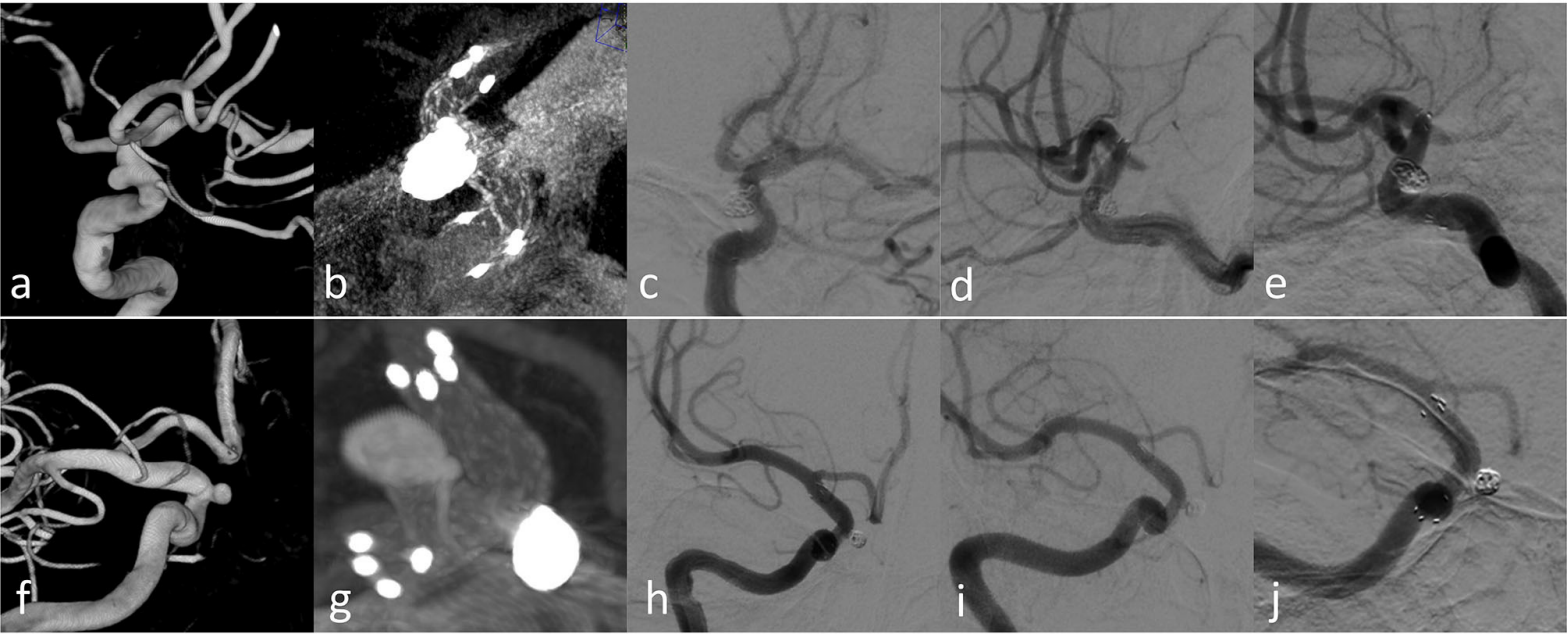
Case 3 (a–e) and Case 12 (f–j) with longer-term radiographic follow-up: (a,f) 3D images before treatment; (b,g) Xper CT immediately after SAC treatment; (c,h) immediate angiography after the endovascular treatment; (d,i) follow-up angiography after 3 months; (f,j) follow-up angiography after 12 months.
